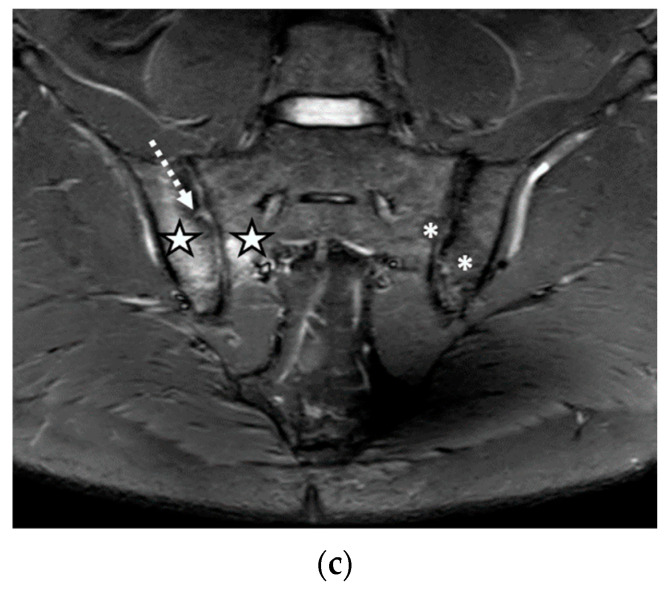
The MRI aspect of the sacroiliac joints included pseudo-widening of both sacroiliac joints, with marked bone marrow edema (star) of both the sacrum and the right iliac bone, in the proximity of the ipsilateral sacroiliac joint space, right iliac bone erosions (discontinuous arrow), bilateral subchondral sclerosis (continuous arrow), and fat metaplasia (asterisk), affecting the left iliac bone, and the left part of the sacrum and backfill (arrowhead) of the left sacroiliac joint space: (a) T1-weighted sequence; (b) T2-weighted sequence (without fat suppression); (c) STIR sequence.
